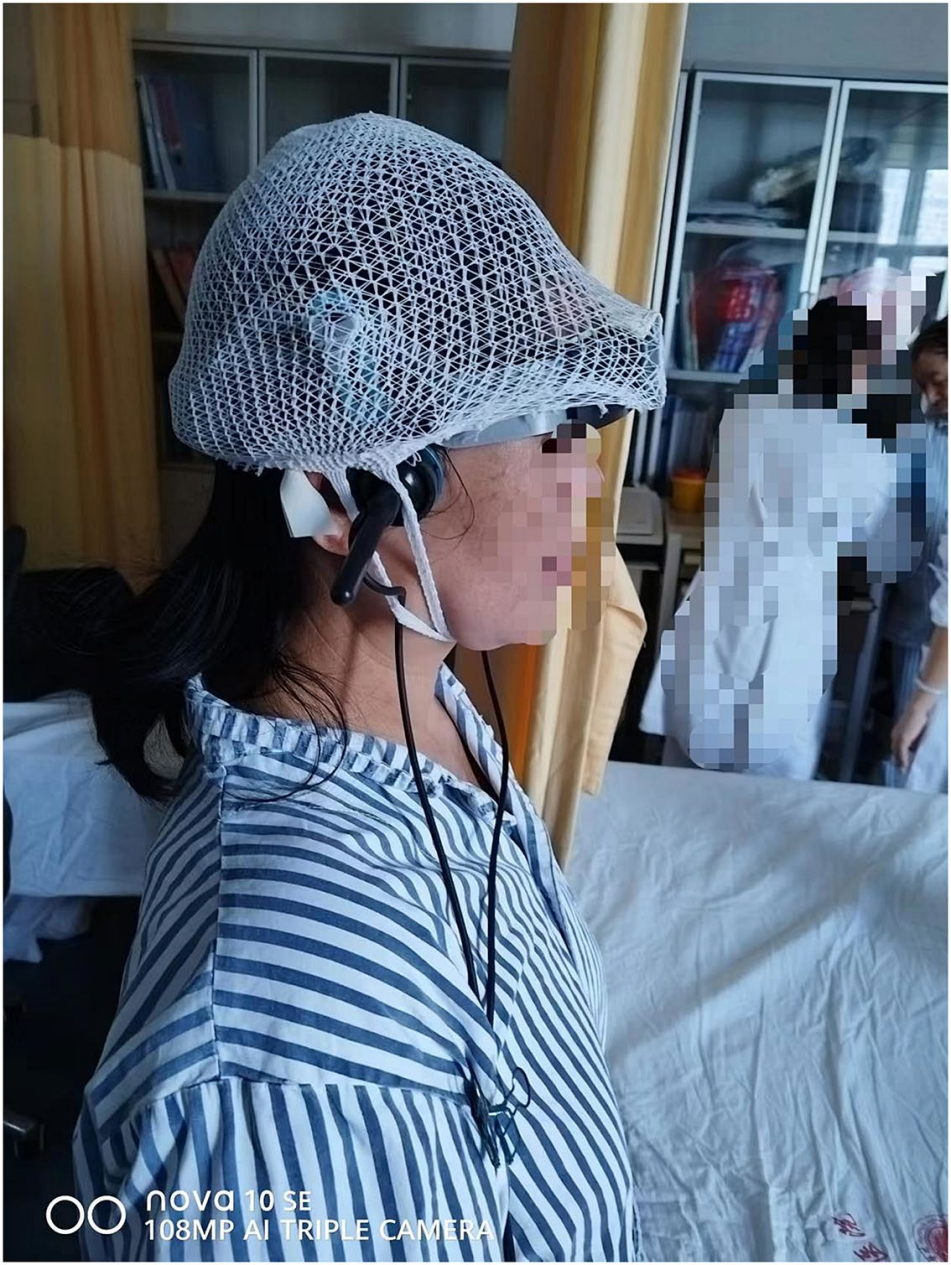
Fixation of the transcranial Doppler (TCD) head frame and probe with a multilayer elastic mesh cap. After confirming the probe position, the multilayer elastic mesh cap is worn on the subject, covering the forehead, probes, and occipital frame of the subject’s head, whereas the handle remains uncovered.
